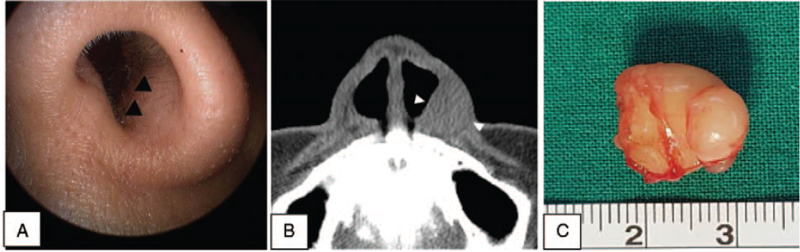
A protrusion of the left vestibule is observed in the endoscopic examination (A). Computed tomography shows a relatively well-circumscribed ovoid mass in the left nasal vestibule (B). A gross picture of the mass shows a 1.5 cm-sized yellowish tumor with a smooth surface (C).
